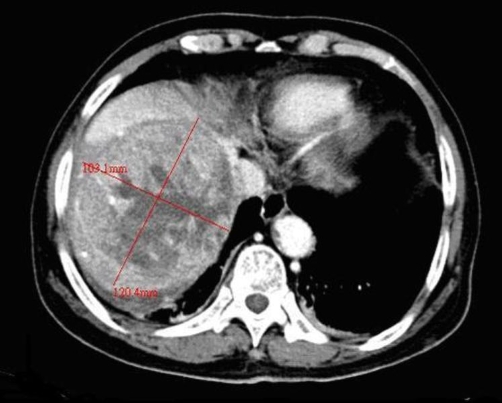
CT scan of a large well-defined mosaic mass lesion in right hepatic lobe with early contrast medium filling and washout with central necrosis, measuring about 12 cm in diameter.
